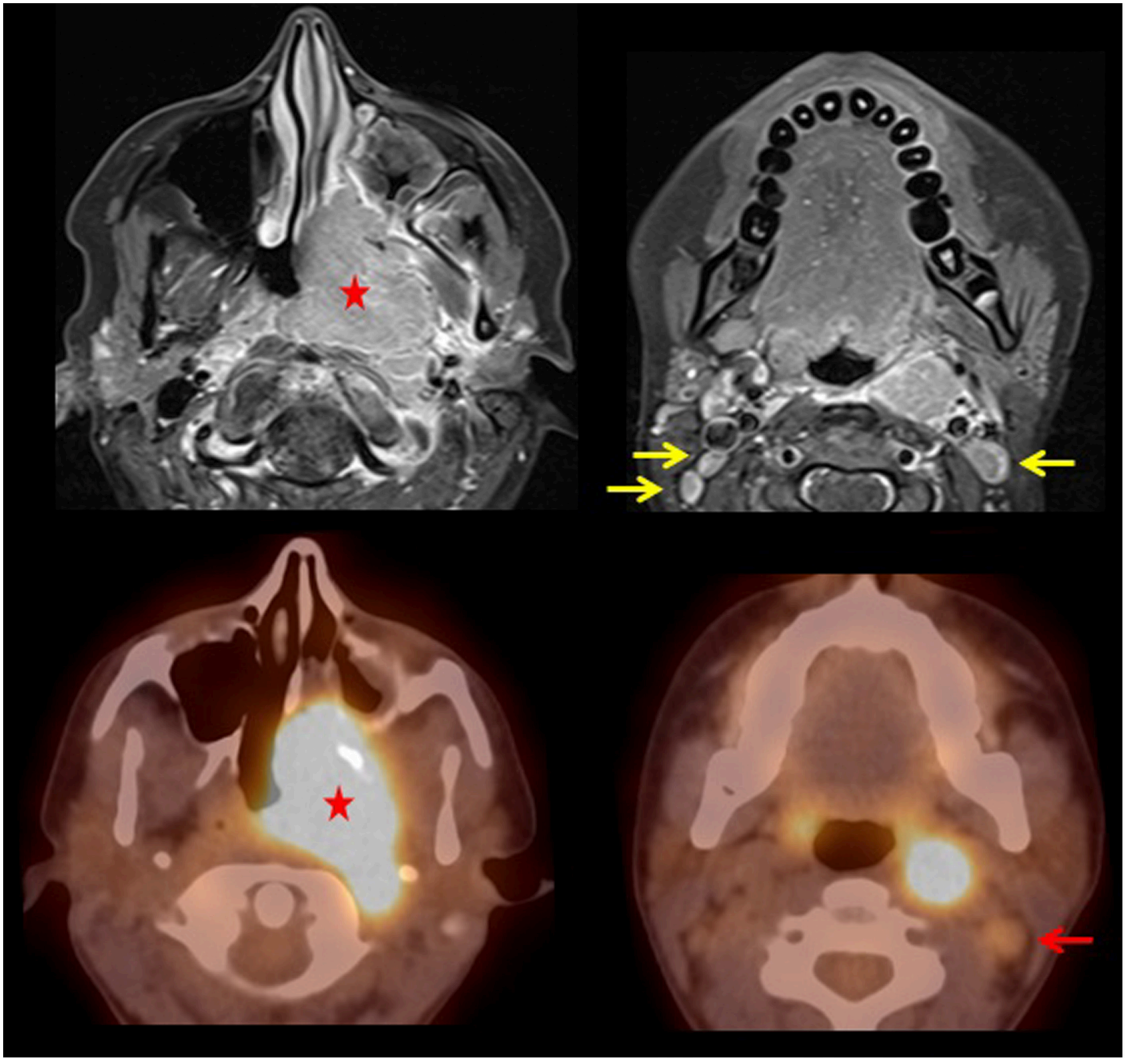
T1 post-contrast fat saturated MRI images (top row) demonstrates diffuse enhancement of the large aggressive mass in the posterior left nasal cavity and nasopharynx (star) invading the left maxillary sinus, nasal septum, left pterygopalatine fossa, left masticator and pharyngeal spaces, and left longus colli muscle causing narrowing of the nasal airway and nasopharynx along with cervical lymphadenopathy (yellow arrows). Fused PET/CT images (bottom row) demonstrate a large FDG avid mass in the left nasal cavity with extension to the maxillary sinus and nasopharynx. There is also an enlarged FDG avid left level 2 cervical lymph node (red arrow).
